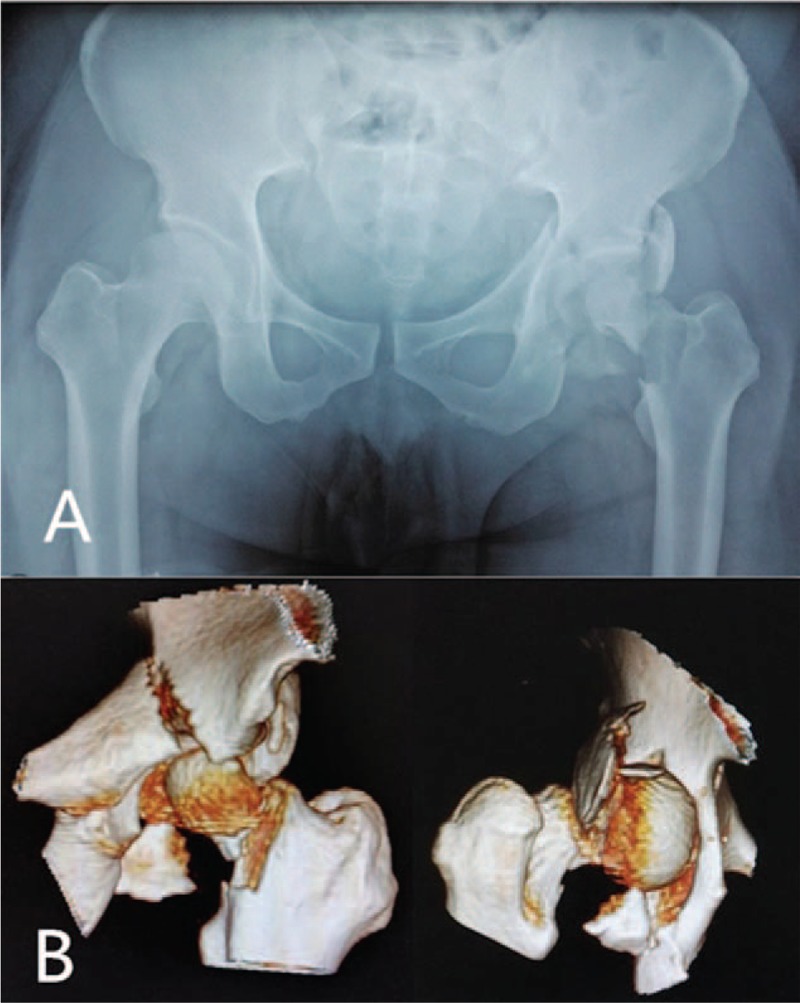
(A) Anteroposterior x-ray image of the pelvis. (B) Anteroposterior three-dimensional computed tomography of hip showing femoral head, femoral neck and transverse acetabular fractures. Posteroanterior three-dimensional computed tomography showing fractures of the femoral neck and posterior wall of acetabulum.
